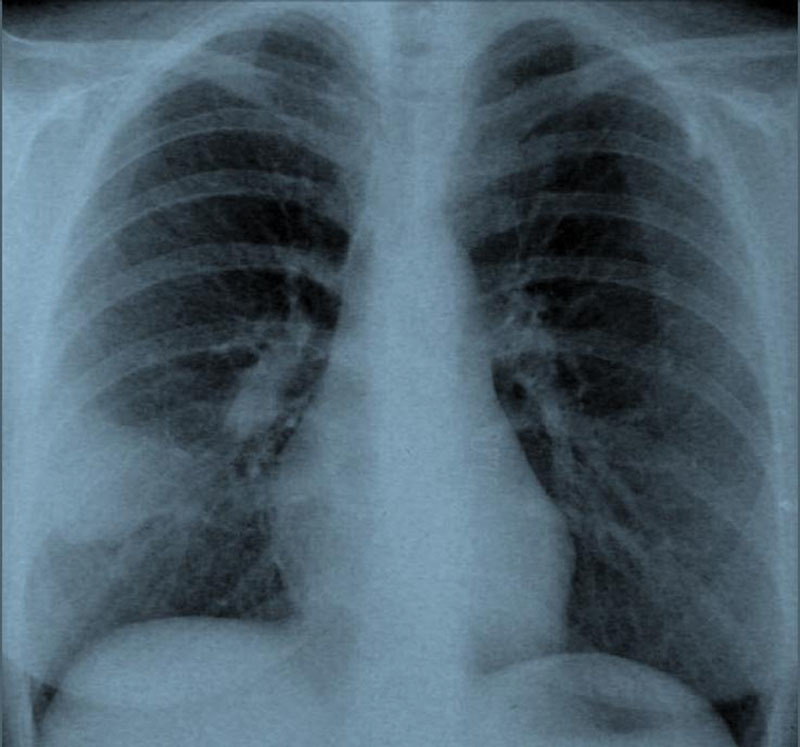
Chest X-ray of the patient at admission to the pulmonology clinic.
